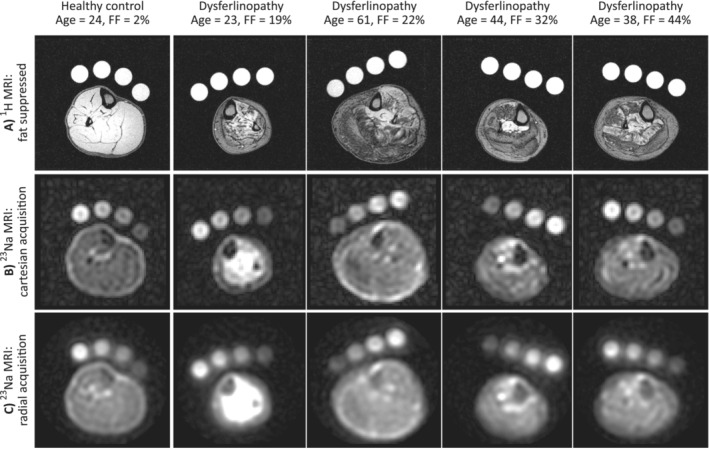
(A) ^1^H‐MR images, (B) ^23^Na‐MR images taken using a cartesian acquisition sequence and (C) ^23^Na‐MR images taken using a radial stack of stars imaging sequence, depicting equivalent image slice positions. An image set from a representative healthy volunteer is presented beside four dysferlinopathy patient image sets, where varying degrees of fat infiltration in the tibialis anterior muscle were measured. ^1^H: hydrogen‐1, ^23^Na: sodium‐23, FF: fat fraction, MRI: magnetic resonance imaging.
